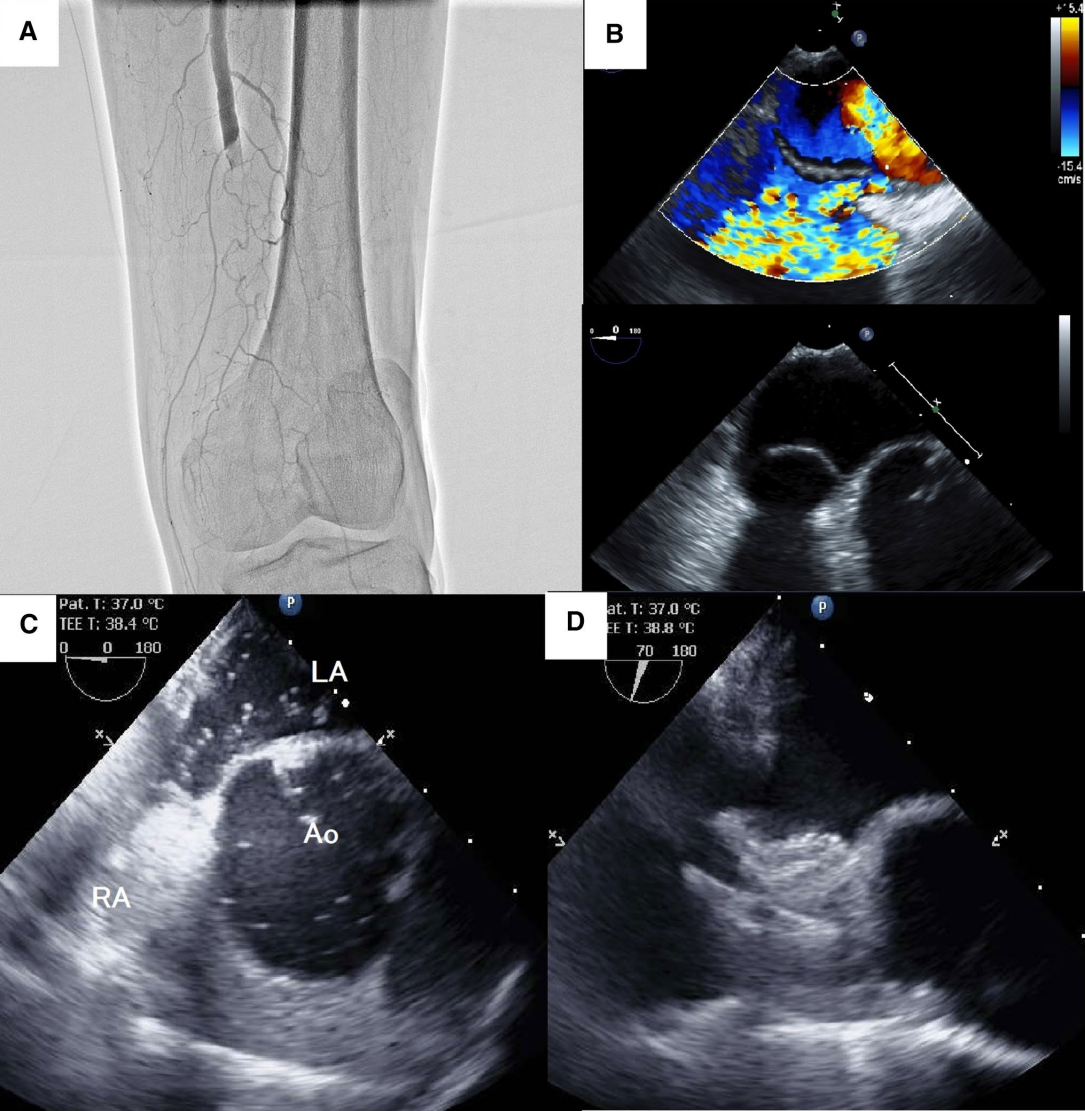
a Angiography of the left leg revealed thrombus in the left superficial femoral artery. b Transesophageal echocardiography showed atrial septal aneurysm and bidirectional shunt between the right and left atrium. c Transesophageal echocardiography showed large number of microbubbles through the patent foramen ovale. d Transesophageal echocardiography showed an Amplatzer Cribriform deployed. *LA* left atrium, *RA* right atrium, *Ao* aorta
